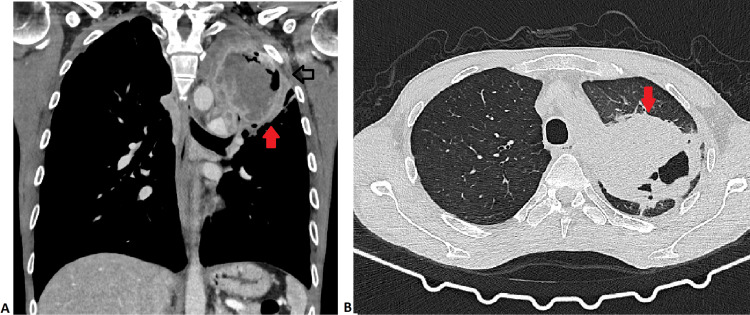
Chest CT scan: (A) coronal, mediastinal window; and (B) axial, lung window. Mass of heterogeneous density in the left upper lobe, predominantly necrotic, with heterogeneous peripheral enhancement and presence of cavitation (red arrow). CT: computed tomography
